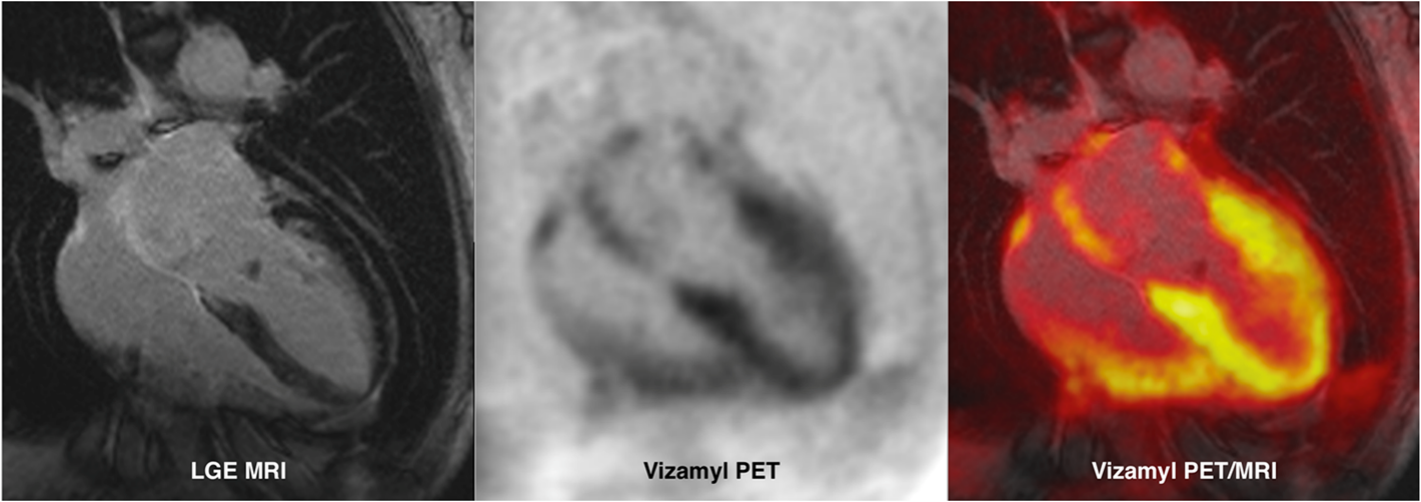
Cardiac amyloid PET/MRI of a patient with hereditary ATTR amyloidosis (case 2): Late gadolinium enhancement (LGE) MRI demonstrates a thickened septal wall, diffuse LGE of the ventricular walls and contrast enhancement of the atria (left panel). On F-18 flutemetamol (Vizamyl) PET scan, moderate to intense tracer accumulation in the right and left ventricular walls as well as in the atria was observed (middle panel). The right panel demonstrates the overlay of amyloid PET and LGE MRI
